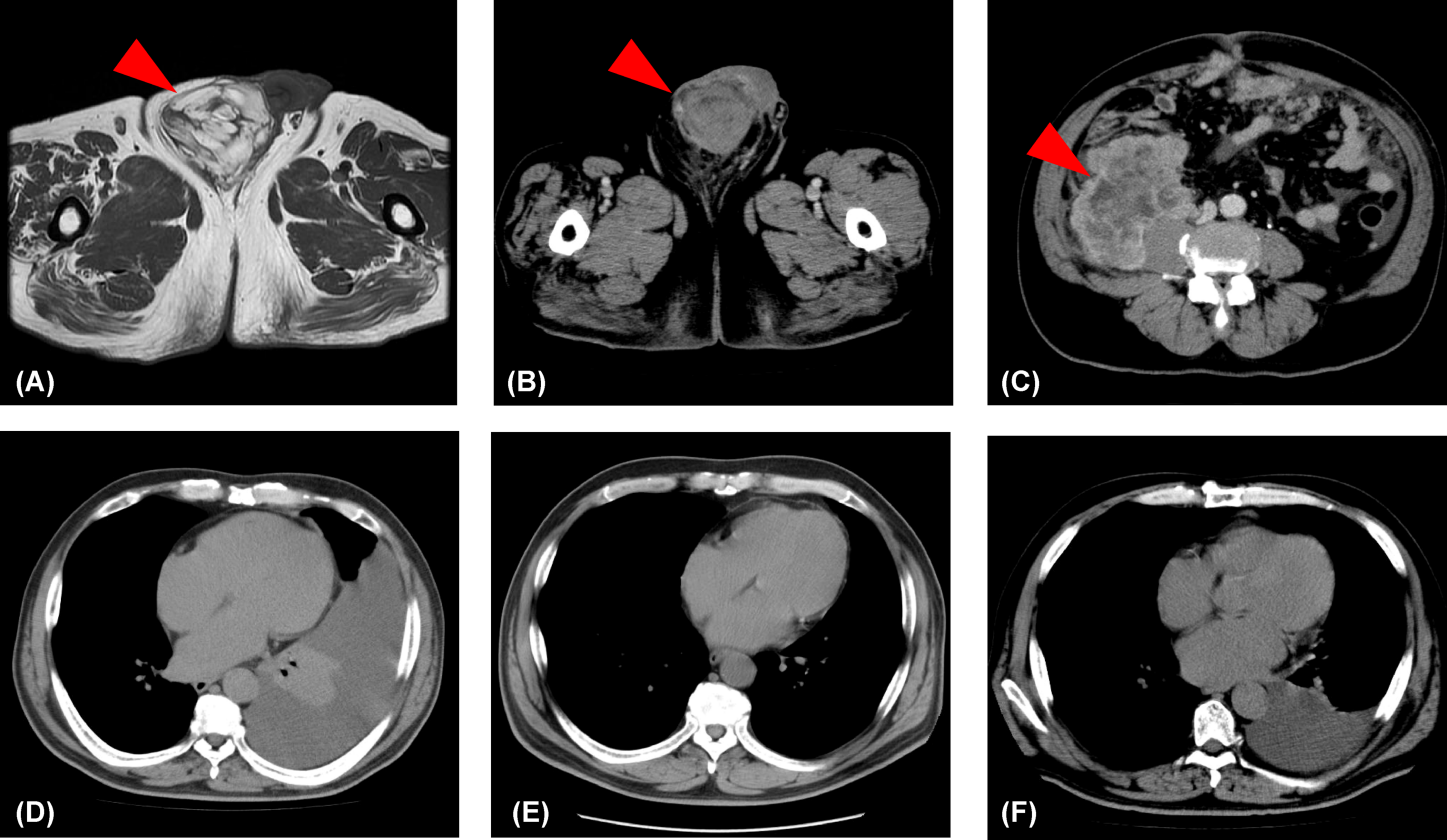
Changes in the lesions identified by imaging. (A) T1-weighted image showing a lipoma (red arrowhead) in the right scrotum. (B) An abdominal-pelvic computed tomography scan showing a substantial mass (>5 cm; red arrowhead) in the right scrotum. (C) An abdominal-pelvic computed tomography scan showing a mass (>10 cm; red arrowhead) in the right retroperitoneum. (D) A 5-week postoperative computed tomography scan showing a left pleural effusion. (E) A computer tomography scan showing no pleural effusion following three cycles of doxorubicin monotherapy. (F) A computer tomography scan showing increased pleural effusion following six cycles of doxorubicin monotherapy.
